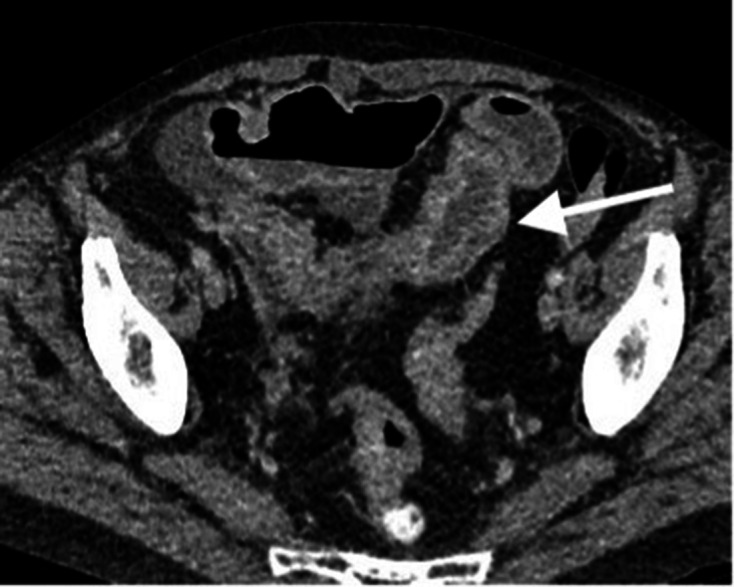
Contrast enhanced CT scan of the abdomen and pelvis in axial plain
